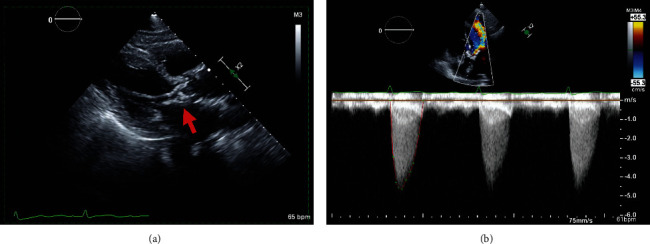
(a) The parasternal long-axis view on transthoracic echocardiography shows bulky calcification of the aortic valve (arrow). (b) The apical five-chamber view on transthoracic continuous-wave Doppler analysis shows a mean gradient of 48 mmHg.
